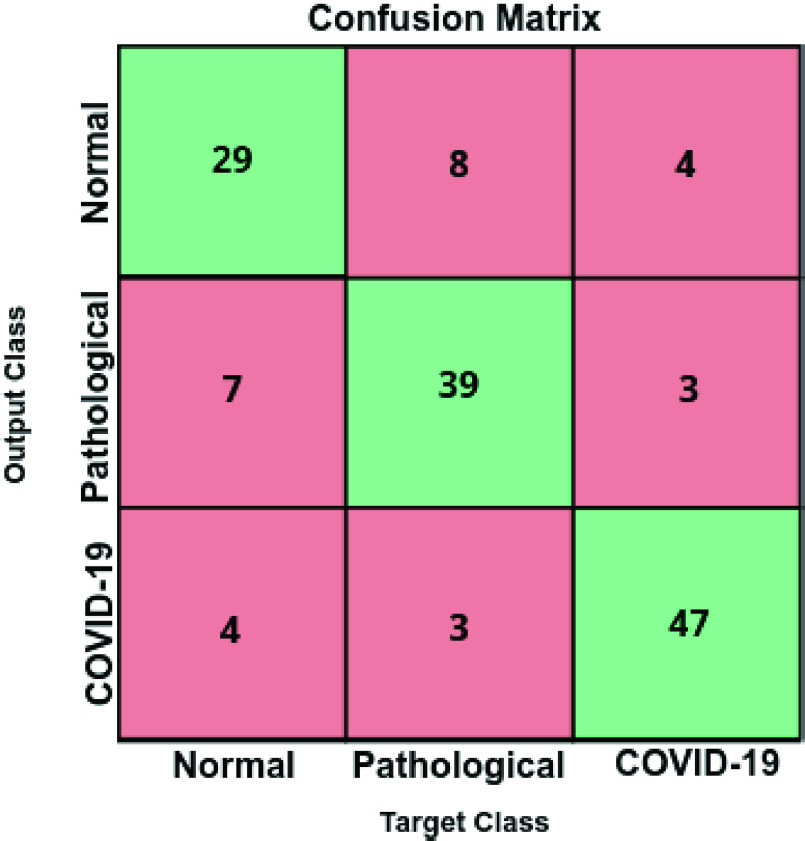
Confusion matrix for the classification of chest X-ray images between Normal vs Pathological vs COVID-19 cases at the test stage.
